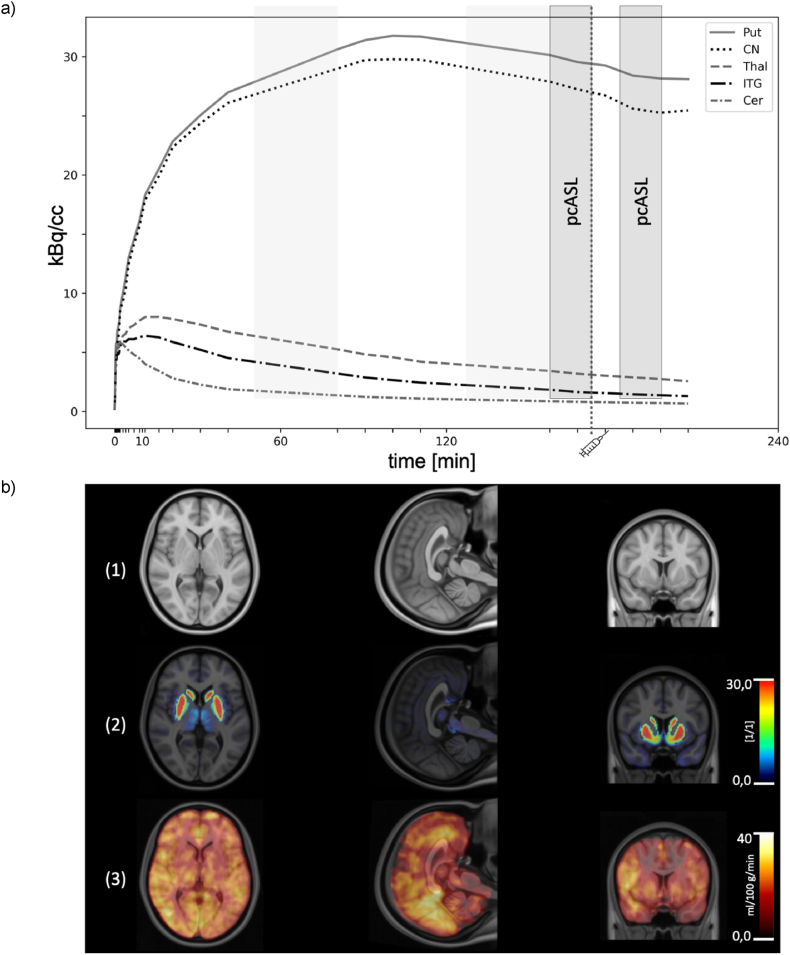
A) Experimental design: Representative time activity curves of the PET acquisition for the bilateral regions: putamen (Put), caudate nucleus (CN), thalamus, inferior temporal cortex (ITC) and cerebellum (Cer). The start of each time frame of the PET acquisition is denoted as tick on the horizontal axis. The time point of the administration of the dopamine agonist apomorphine is depicted as vertical dotted line. Periods of the perfusion weighted image acquisitions (ASL) are marked in dark grey. Inter-session pauses of PET-acquisition are marked in light grey, the signal of the inter-session pauses is based on linear interpolation. b) Representative parametric maps fused with a structural T1-weighted MR MNI152 NLIN 6th generation template (1), fusion parametric map of the dopamine receptor D_2/3_ binding potential combined with the structural T1-weighted MR MNI152 template (2) and fusion parametric map of cerebral blood flow combined with the structural T1-weighted MR MNI152 template (3).
